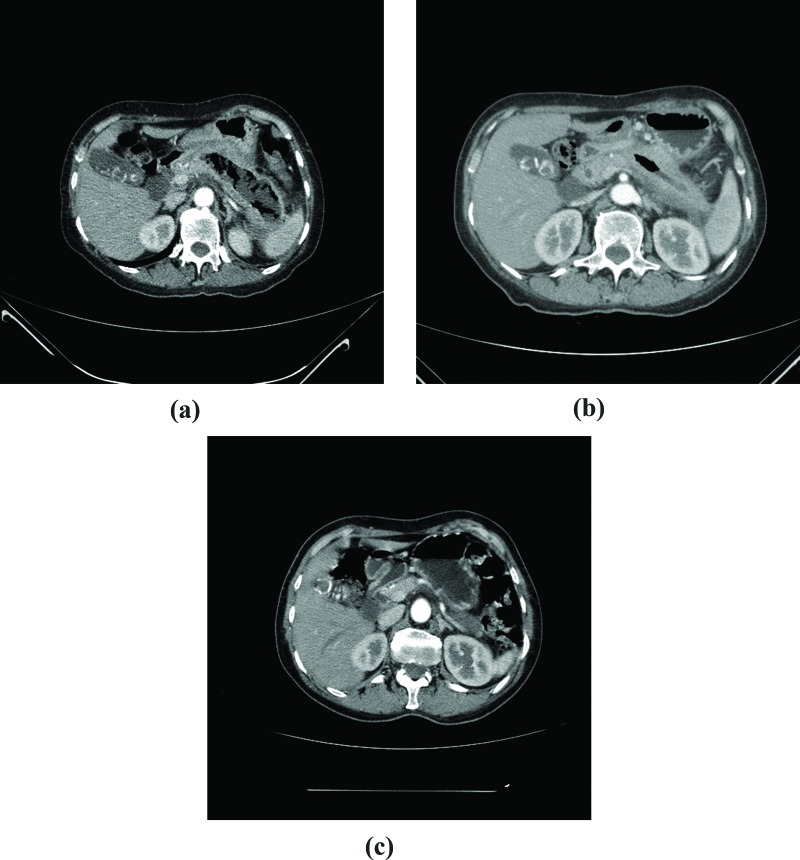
CT scan, case 3 A: First exam, 3 weeks after the onset of symptoms: pancreatic necrosis with gas throughout the body and the tail of the pancreas. B: After 50 days of follow-up: significant necrosis and gas are still present. C: 3 months after hospital discharge: no pancreatic necrosis or collection.
